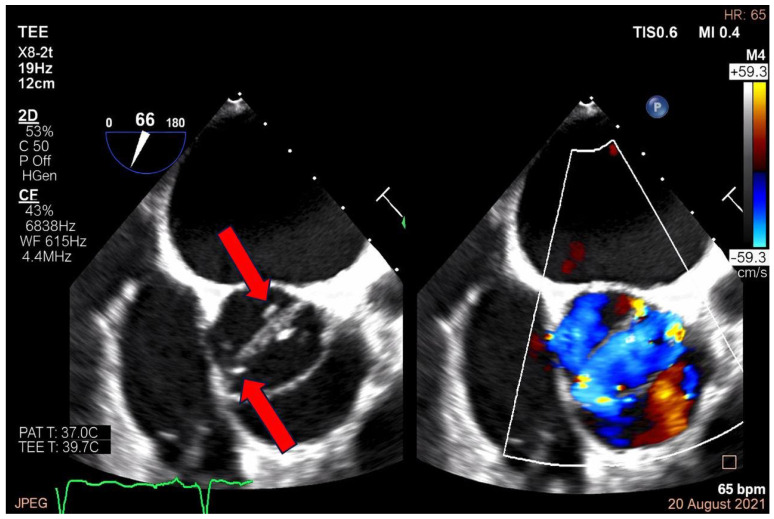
Transesophageal echocardiography images demonstrating valvular involvement in infective en-do-carditis. Left panel: two-dimensional TEE view showing irregular echogenic masses attached to the valve leaflets (red arrows), consistent with valvular vegetations. Right panel: color Doppler imaging demonstrating turbulent regurgitant flow across the affected valve. In the color Doppler map, red indicates blood flow toward the transducer and blue indicates flow away from the transducer, while the mosaic pattern (red–blue–yellow) reflects turbulent flow, suggestive of significant valvular regurgitation associated with the infectious process.
